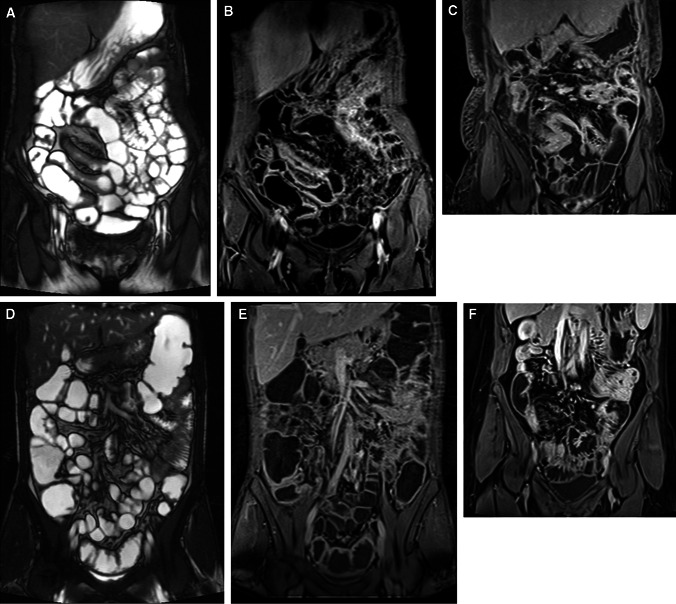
A T2-weighted sequence showing thickening of bowel wall before anti-tumor necrosis factor therapy (A). Dynamic contrast enhanced T1-volume interpolated gradient-echo sequence showing thickening of the bowel wall with layered enhancement, fat wrapping with a proliferation of mesenteric vasculature (B) and with enlargement of mesenteric lymph nodes before starting biological treatment (C). B T2-weighted sequence showing a significant decrease of bowel wall thickening after induction anti-tumor necrosis factor therapy (D). Dynamic contrast enhanced T1-volume interpolated gradient-echo sequence showing a significant decrease of bowel wall thickening without pathological enhancement, fat wrapping with a proliferation of mesenteric vasculature are not present after finishing induction biological treatment (E). The diameter of enlarged mesenteric lymph nodes decreased significantly after the therapy (F).
